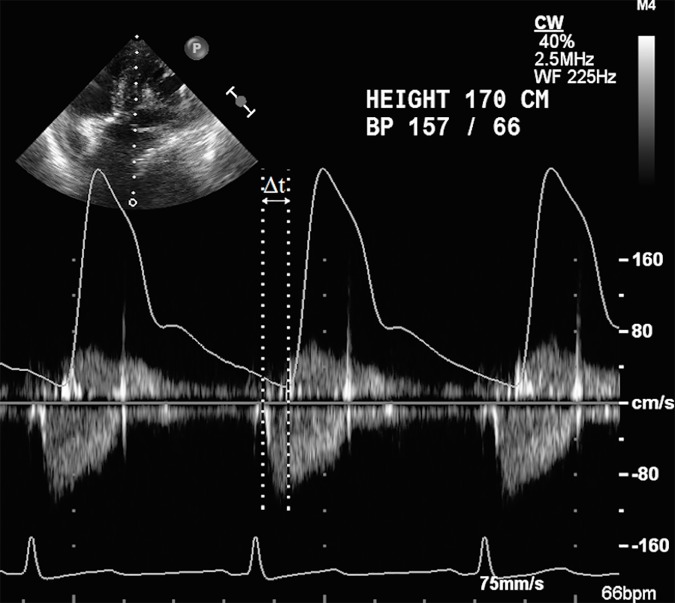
Representative image of measurements. We measured the pulse transit time (Δt) from the start of ejection on continuous wave Doppler waveform to the upstroke on the radial arterial waveform, and left ventricular outflow tract velocity time integral by tracing the continuous wave Doppler waveform.
